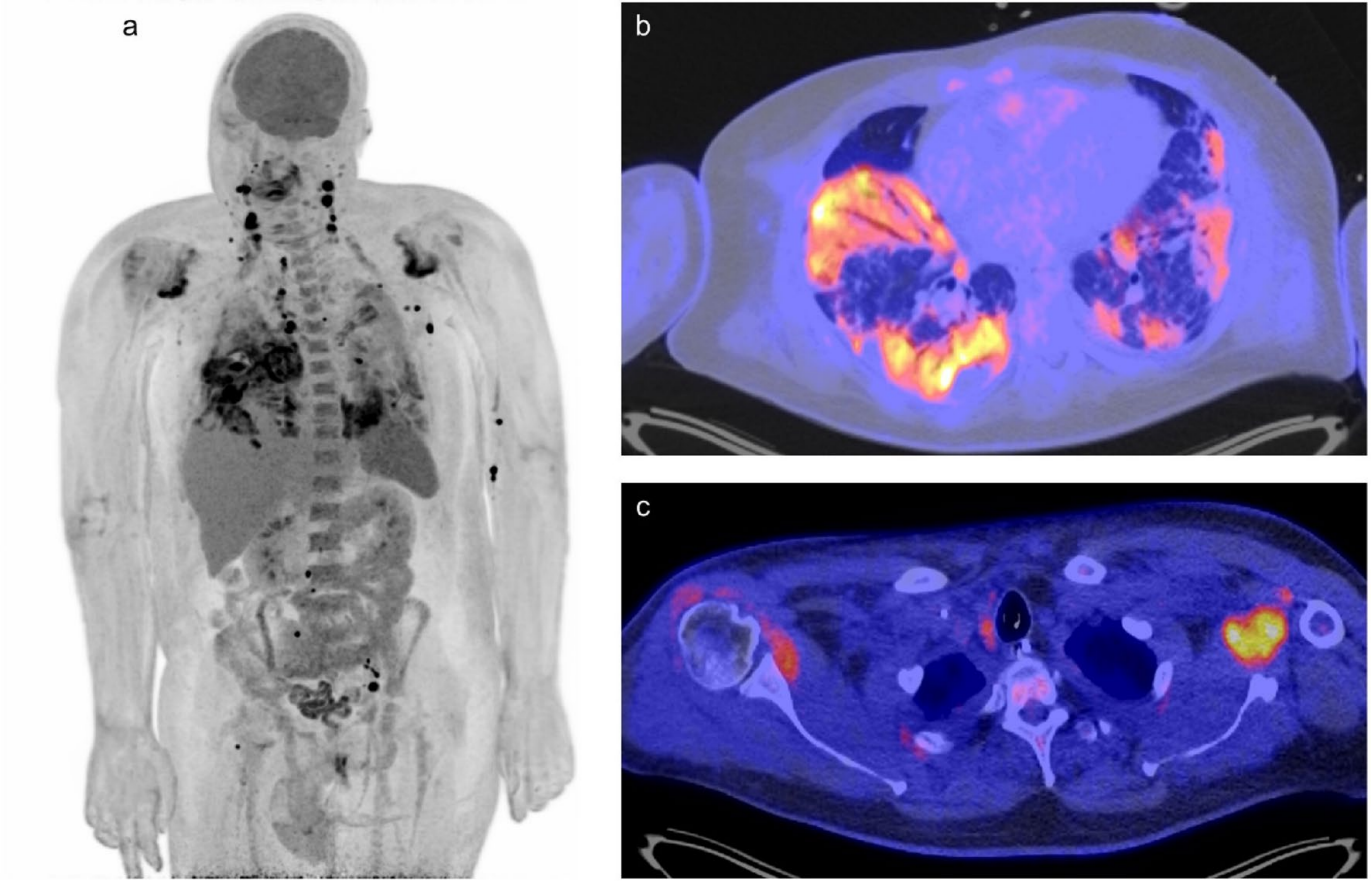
[^18^F]FDG-PET/CT of patient 2. A 46-year-old male was admitted to the ICU 1 week after developing symptoms of COVID-19. Due to ongoing inflammation without focus and deteriorating multiorgan failure, an [^18^F]FDG-PET/CT was performed to evaluate the presence of a secondary infection. No infection focus was found and the patient stayed SARS-CoV-2 positive till his dead. a Maximum intensity projection. Physiological uptake in the liver and intestines. Atypical low intensity in the kidneys due to kidney failure. Reduced uptake in the brain due to sedation. Reactive uptake in the spleen and bone marrow. The lymphadenopathy can be seen very clearly. b Transaxial pulmonary fusion PET/CT image with [^18^F]FDG uptake in the peripheral and central areas of the lung. CT in lung setting. c Transaxial fusion PET/CT image of the shoulder regions with [^18^F]FDG uptake in the anterior medial muscles of the shoulders (left more than right). CT in soft tissue setting. *SUV-bw threshold of 5.00; α* = *50%*
